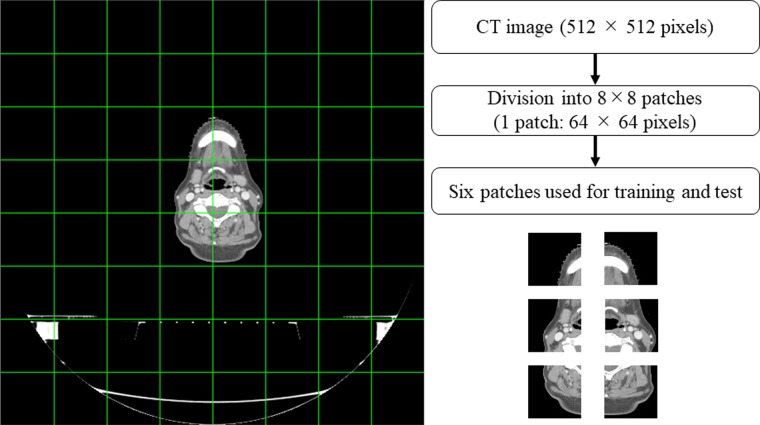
Creation process of the image patch from an original CT image.
